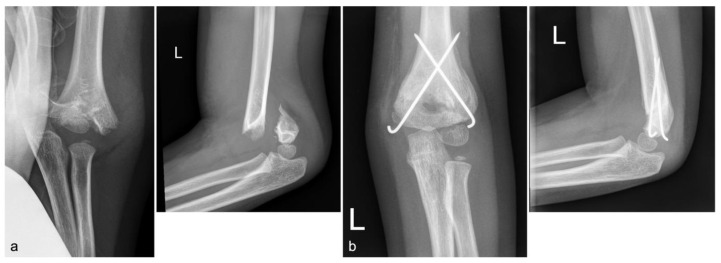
The course of a surgically treated type IV supracondylar fracture of the left (L) arm of a 6-year-old boy who fell from a climbing frame (1.5 m). (a) Initial a.p. and lateral X-rays showing the type IV fracture according to the Von Laer classification. The boy was operated on the same day. (b) A.p. and lateral X-rays after bilateral K-wire fixation 6 weeks postoperatively with new bone formation. Implant removal was performed 7 weeks after the initial surgery. The patient had no deficits 4 weeks after implant removal.
